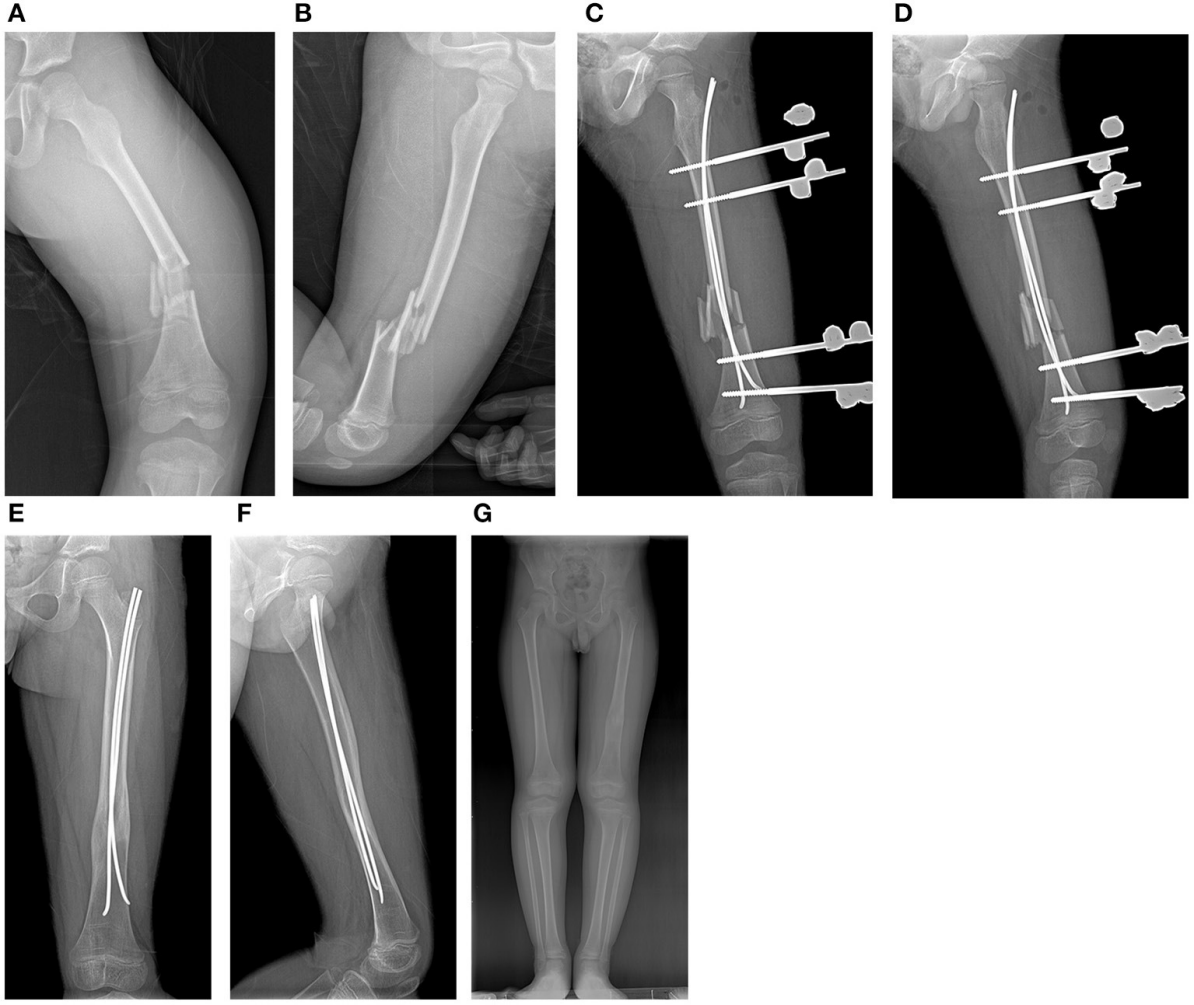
Case 10. (A,B) Comminuted type IV femur fracture in an 8-year-old boy; (C,D) postoperative radiographs; (E,F) removal of EF; (G) final outcome.
